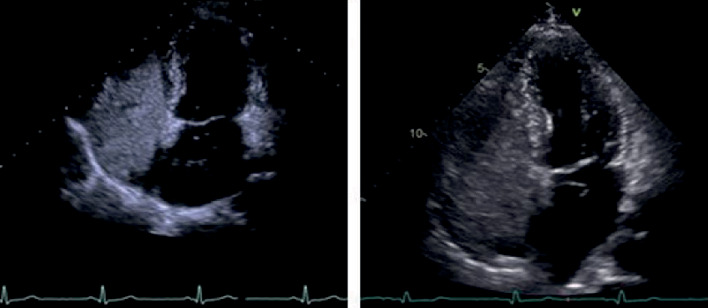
Contrast transthoracic echocardiographic (cTTE) images of PFO, clearly showing the interatrial septum and the agitated saline contrast.
